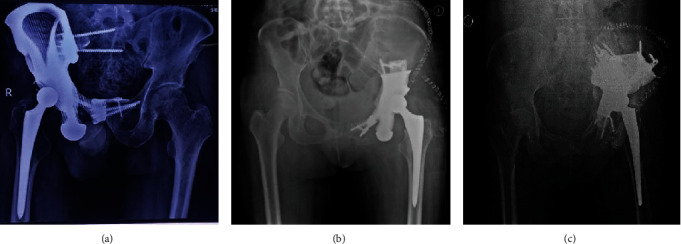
Postoperative X-ray images. (a) Prosthesis for regions I+II+III. (b) Prosthesis for regions II+III. (c) Prosthesis for regions I+II.
